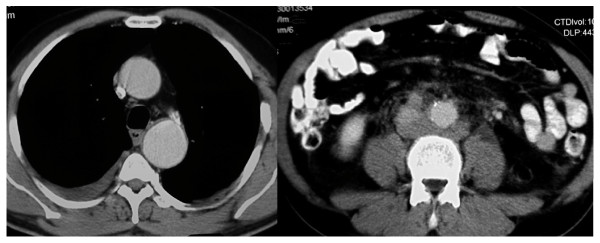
Contrast enhanced CT-scan at the time before onset of chemotherapy. 1a: Chest CT. patent aorta, no thrombotic deposits. 2a: abdominal CT: patent aorta, no thrombotic deposits. Minor calcifications in the aortic wall, no major atherosclerotic signs. Note: metastatic deposits around the abdominal aorta; larger metastatic mass located cranial to this section (not shown).
